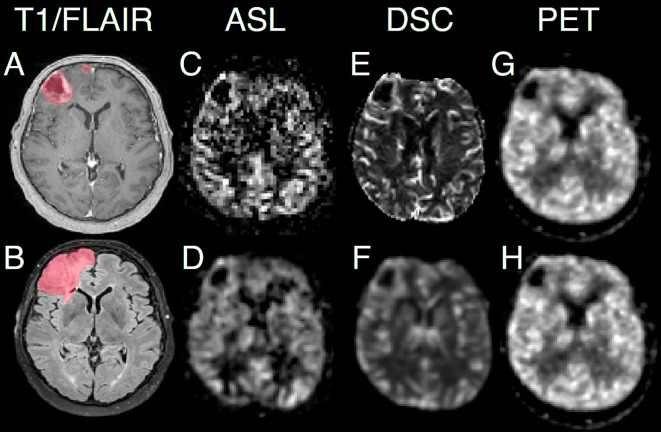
Single transverse slice for a representative case (participant 2, glioblastoma, IDH wild-type, male, 66y). (A) post-gadolinium T1-weighted image with overlaid delineated lesion and (B) T2-FLAIR image with overlaid segmented edema; (C, D) arterial spin labeling (ASL), (E, F) dynamic susceptibility contrast (DSC), (G, H) positron emission tomography (PET) images. The top row (A– D) shows the original images in native resolution for similar slice positions. The bottom row (E–H) shows the aligned images smoothed to the common 5.5x5.5x5.5 mm^3^ resolution. ASL, arterial spin labeling; DSC, dynamic susceptibility contrast; GBM, glioblastoma.
